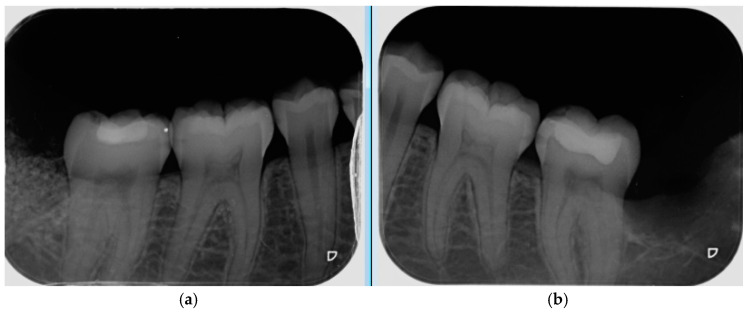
Intra-operative radiographs taken after autogenous dentin graft placement on study side (a) and Spontaneous healing with blood coagulate on control side (b).
